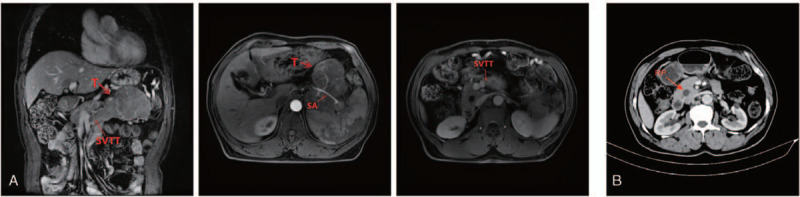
MRI of the abdomen. (A) Preoperative MRI: The mass at the distal end of the pancreas is shown by an axial view and a coronal view. (B) Postoperative MRI: no signs of recurrence were found. RP = residual pancreas, SA = splenic artery, SV = splenic vein, SVTT = splenic vein tumor thrombus, T = Tumor.
